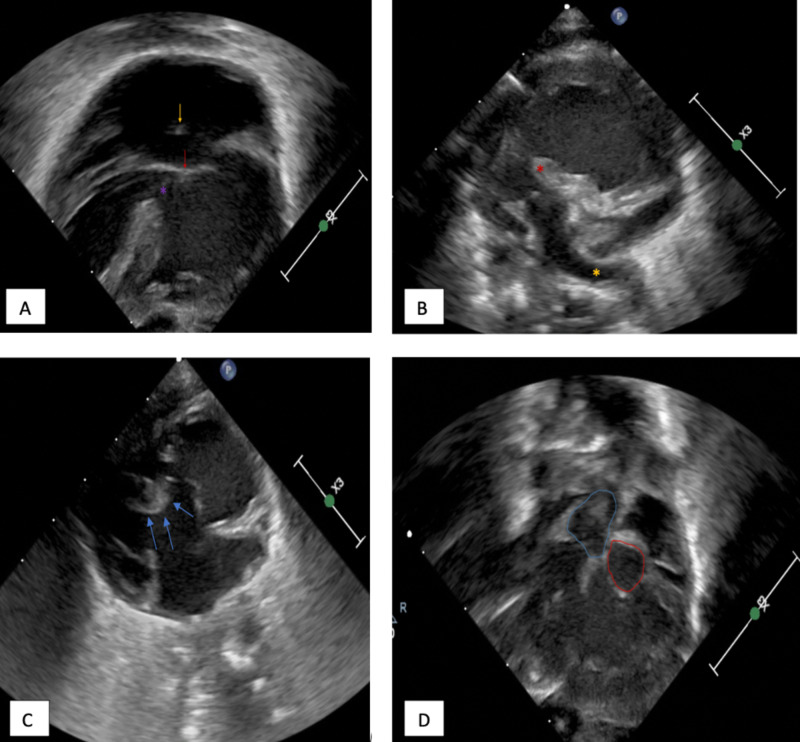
A: Ultrasonography apical 4-chamber view of the heart. There is a common atrium with small intact septum (yellow arrow), common atrioventricular valve (red arrow), and ventricular septal defect (purple asterisk). B: The main pulmonary artery originates from the left ventricle with the left pulmonary artery clearly visualized (yellow asterisk). The infundibulum is labelled with red asterisk. Sub-pulmonary stenosis is also noted. C: Visualization of the patient’s left-sided ventricle. The attachment of valve leaflet to ventricular septum (blue arrow) is further confirmation of right ventricle. D: Ultrasonography right ventricular outflow tract view. There is a double outlet right ventricle, which gives rise to the pulmonary trunk and aorta.
